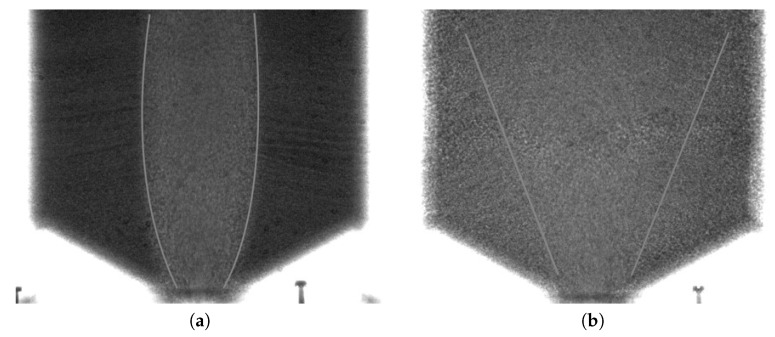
Radiography images of funnel flow in the silo: (a) initially dense packing; (b) initially loose packing. Contour lines indicate approximate flow funnel shape.
